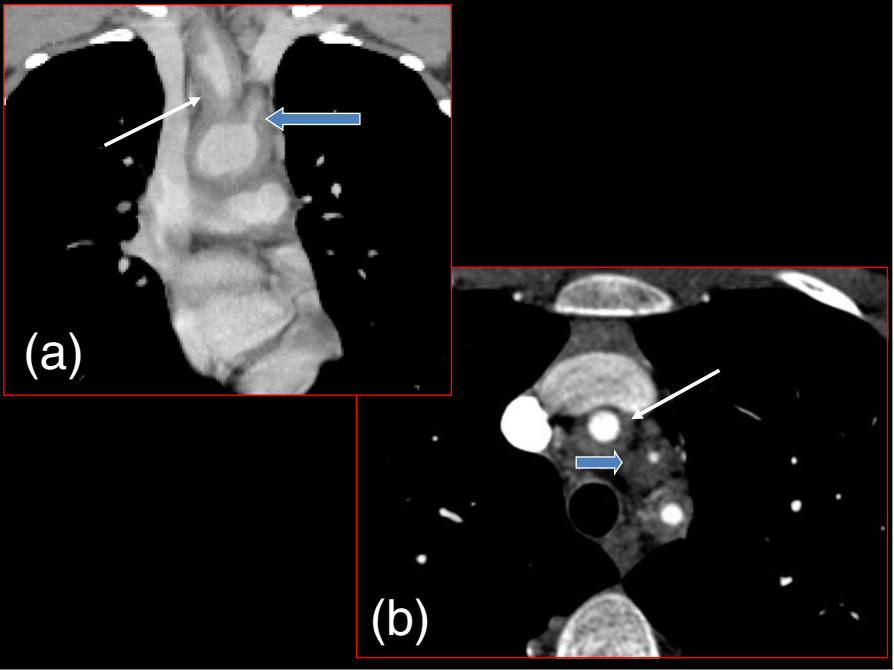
Coronal (a) and Axial (b) CT Chest. Concentric wall thickening at the origin of the major aortic arch branches: brachio-cephalic trunk (thin white arrow) and left common carotid artery origin (thick blue arrow).
